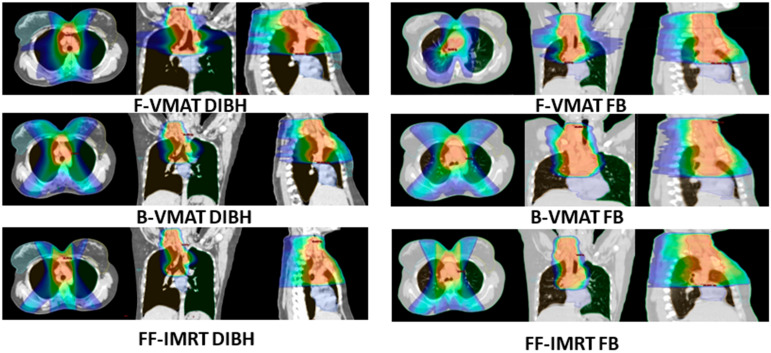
Dose color wash of the representative patient in axial, coronal sagittal CT images regarding three planning techniques used alongside DIBH and FB breathing methods. The volume receiving 5 Gy is indicated by blue color The green color wash represents the volume receiving 17.5 Gy (70%), while the orange color dose wash represents the volume receiving 23.9 Gy (95%). F-VMAT: full arc-volumetric modulated arc therapy; B-VMAT: butterfly volumetric modulated arc therapy: FF-IMRT: fixed field intensity-modulated radiation therapy; DIBH: deep inspiration breath hold; FB: free breathing.
